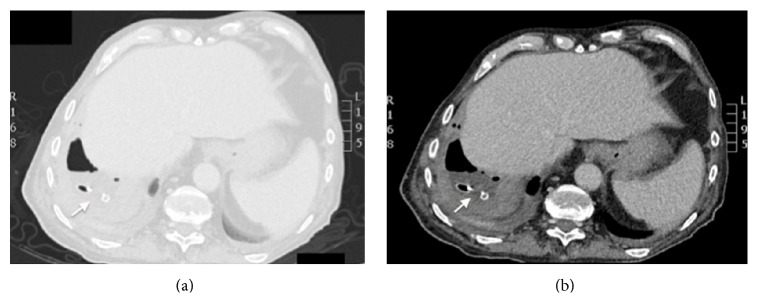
Computed tomography. Pigtail catheter correctly placed in the right lower lobe.
